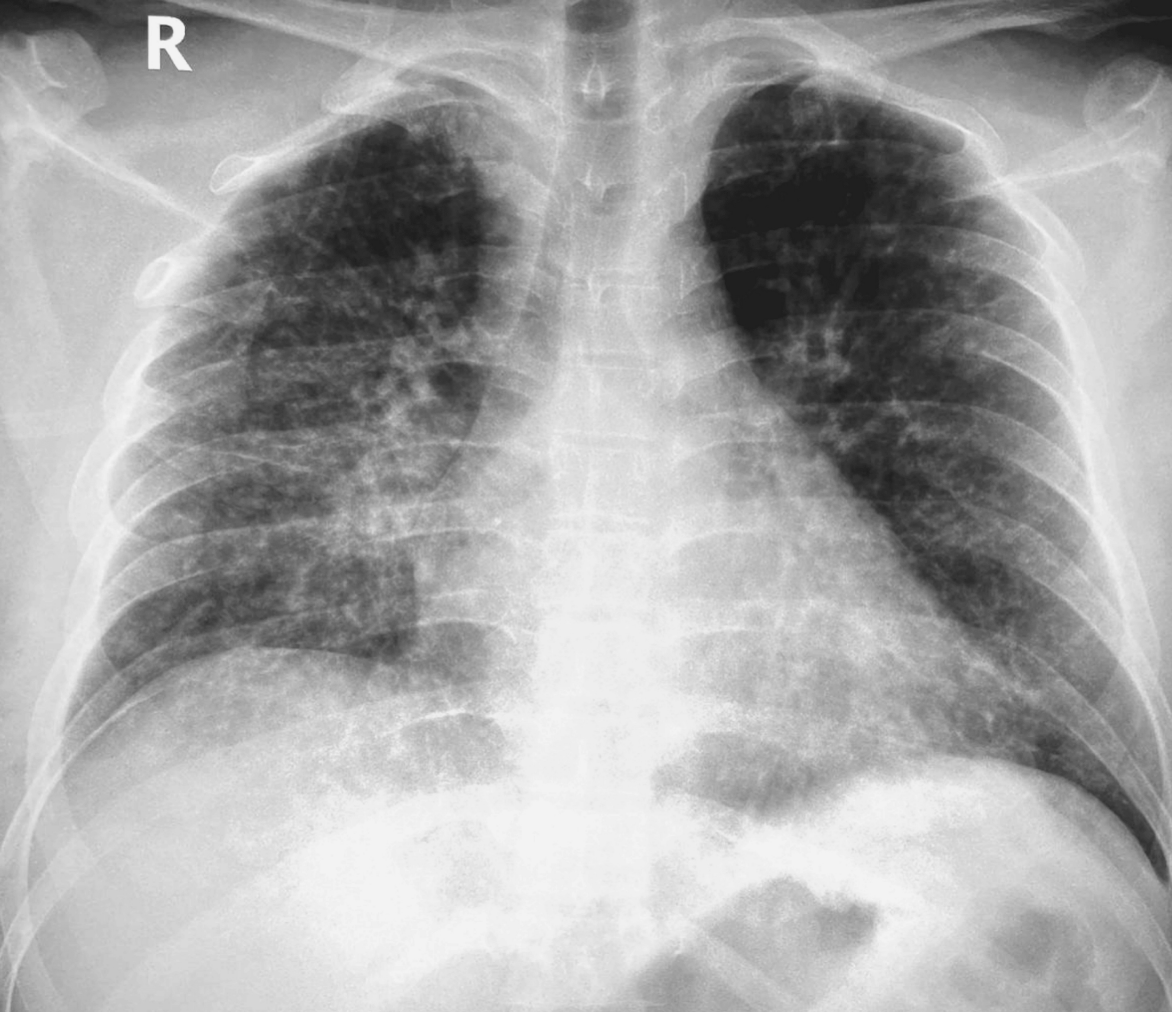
Chest radiograph of Case B The image shows ground glass opacities and infiltrations bilaterally
